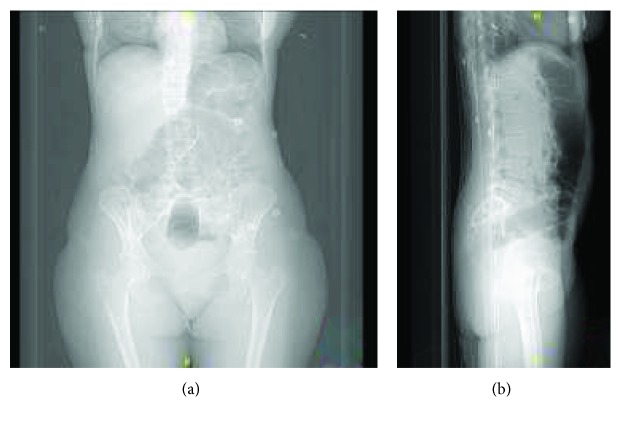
Standard frontal (a) and lateral (b) radiographs reveal the foreign body.
